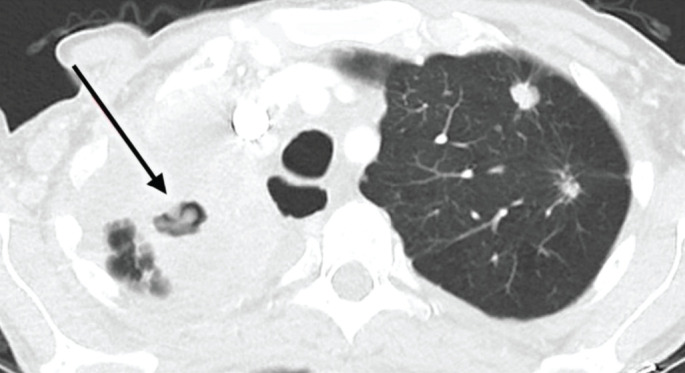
Computed tomography scan showing progressive tuberculosis disease (arrow) in the right lung of a cancer patient treated with nivolumab. Reproduced from [45] with permission.
